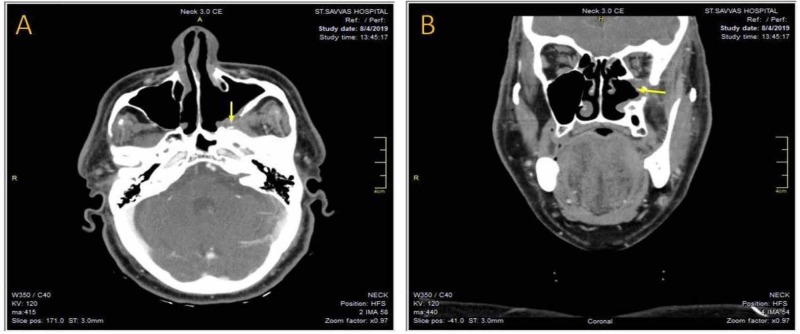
(A) The axial and (B) the coronal views of the postoperative CT scan of the sinuses after 18 months, showing the healed posterior wall of the left maxillary sinus and the left pterygopalatine fossa free of pathology.
